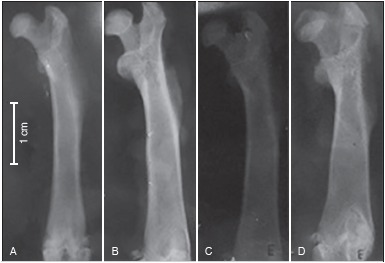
Radiographs of femurs. A: IntSed (control); B: IntTr; C: OVXSed; D: OVXTr.
